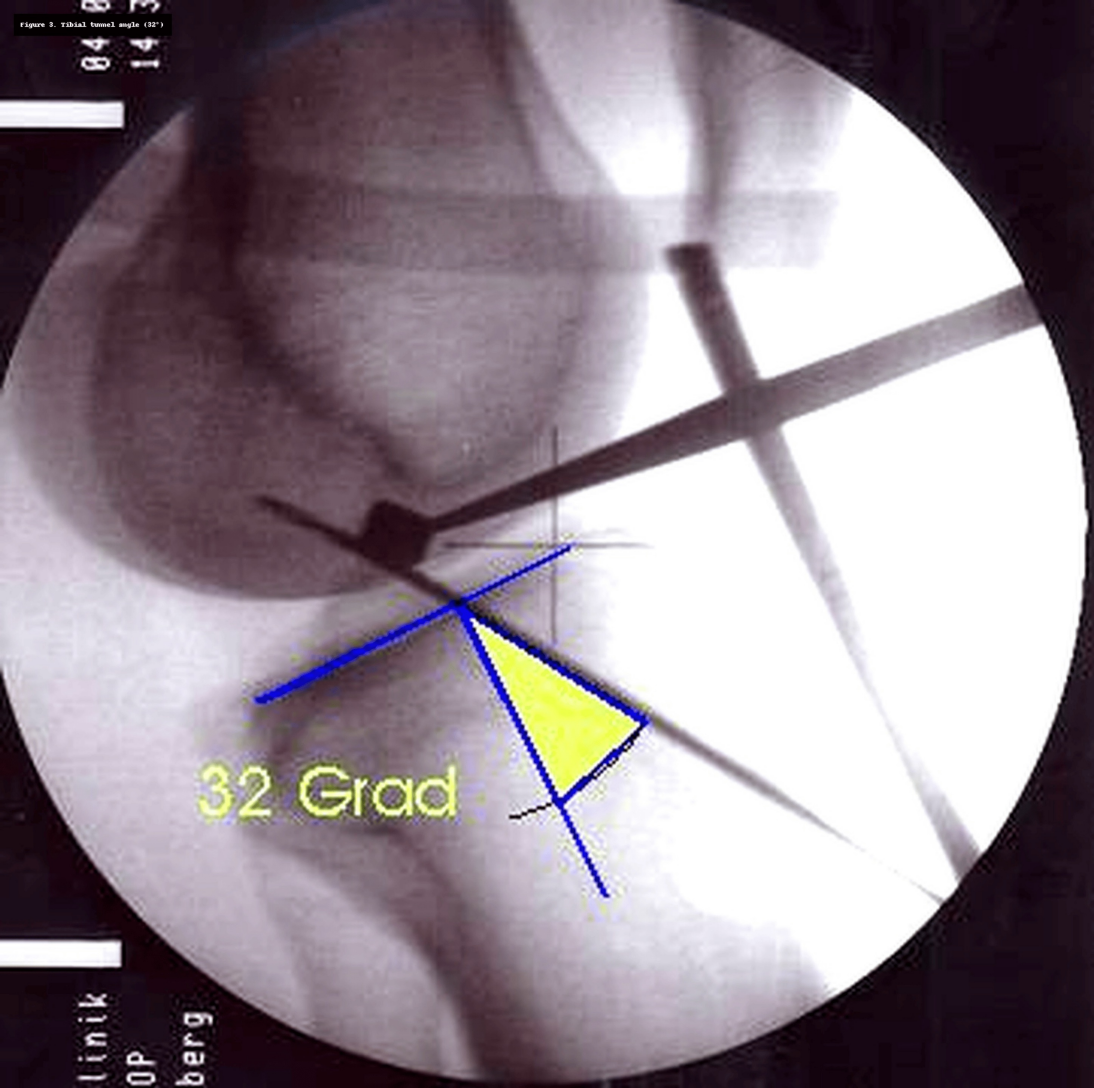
Radiographic measurement of the tibial tunnel angle in the sagittal plane (32°).
